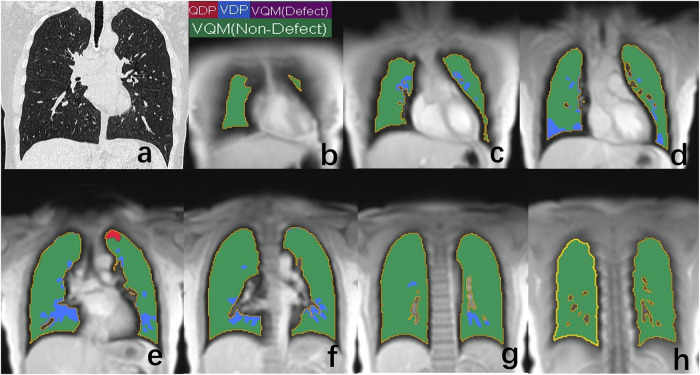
LDCT and PREFUL MRI ventilation/perfusion maps of a 66-year-old healthy male. a The coronal LDCT. b–h The coronal PREFUL MRI Ventilation/Perfusion maps of the whole lung. In ventilation/perfusion maps, red areas indicates QDPexclusive (percentage of areas with perfusion defects but without ventilation defects = 0.3%), blue areas represents VDPexclusive (percentage of areas with ventilation defects but without perfusion defects = 6.6%), green areas corresponds to VQMnon-defect (percentage of areas without perfusion defects or ventilation defects = 93.1%), and purple areas denotes VQMdefect(Percentage of areas with concurrent perfusion and ventilation defects = 0.0%). PREFUL MRI, phase-resolved functional lung magnetic resonance imaging
